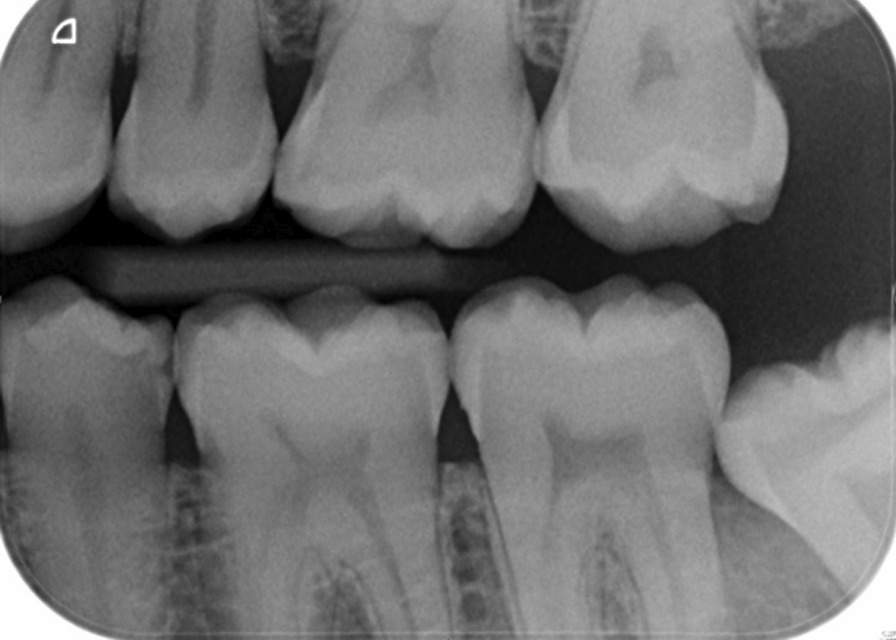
Left bitewing radiograph of impacted and partially erupted mandibular third molar contacting the adjacent second molar below the CEJ
